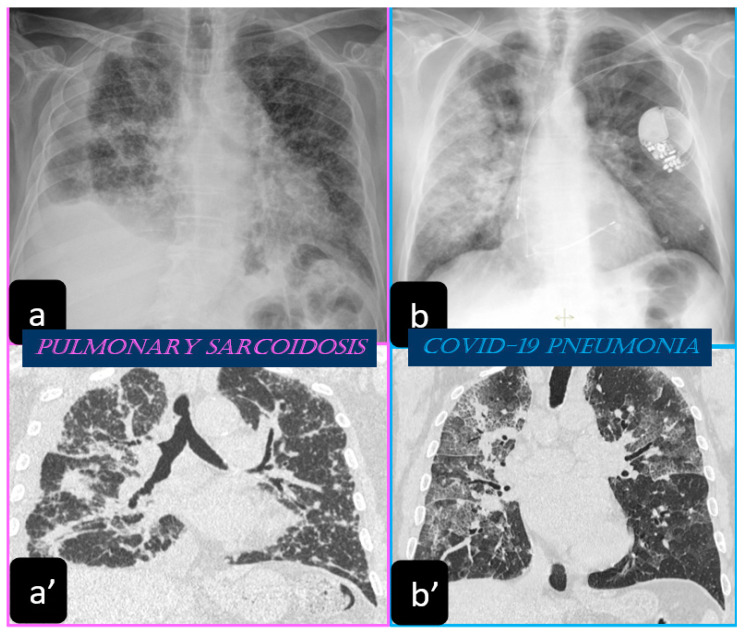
Chest X-ray of patients with pulmonary sarcoidosis shows the typical symmetric reticulonodular opacities (stage III). On the left side, the chest X-ray (a) and coronal (a’) high resolution computed tomography (HRCT) scans show the typical symmetric reticulonodular opacities of sarcoidosis (stage III). On the right side, the chest X-ray (b) shows the bilateral consolidation and ground glass haze. The coronal (b’) HRCT scan shows the presence of consolidation and ground-glass opacity with a superimposed interlobular septal thickening (“crazy-paving” pattern).
